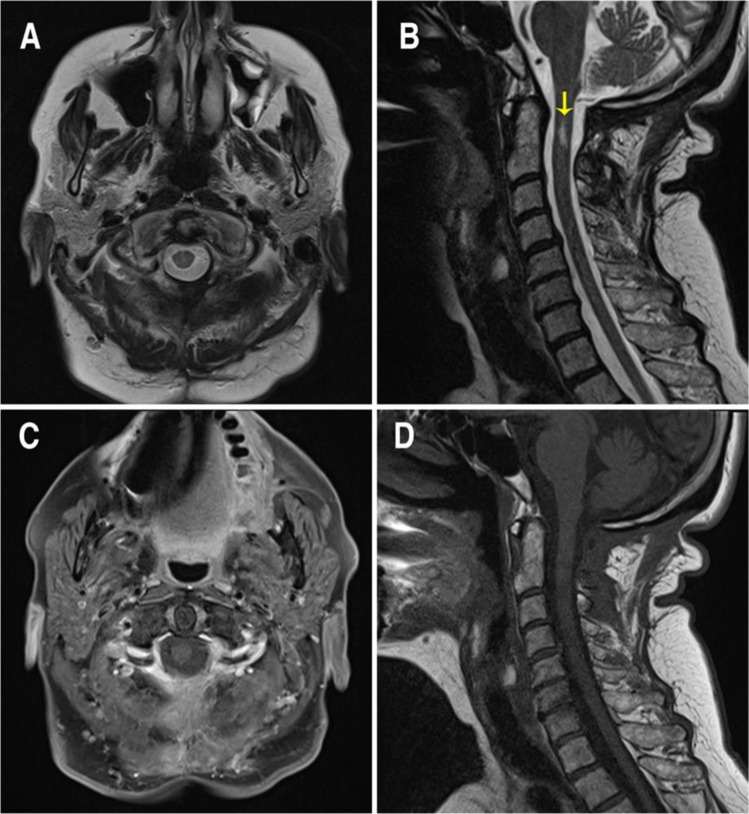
Three-month postoperative MRI. A Coronal T2-weighted MRI view showing absence of tumor recurrence and spinal cord decompression. B Sagittal T1-weighted MRI view showing signal alteration at the C1–C2 level of the spinal cord, consistent with persistent myelopathy (yellow arrow). C Coronal T1-weighted MRI view. D Sagittal T1-weighted MRI view showing signs of C1 hemilaminectomy and C2 partial laminectomy
